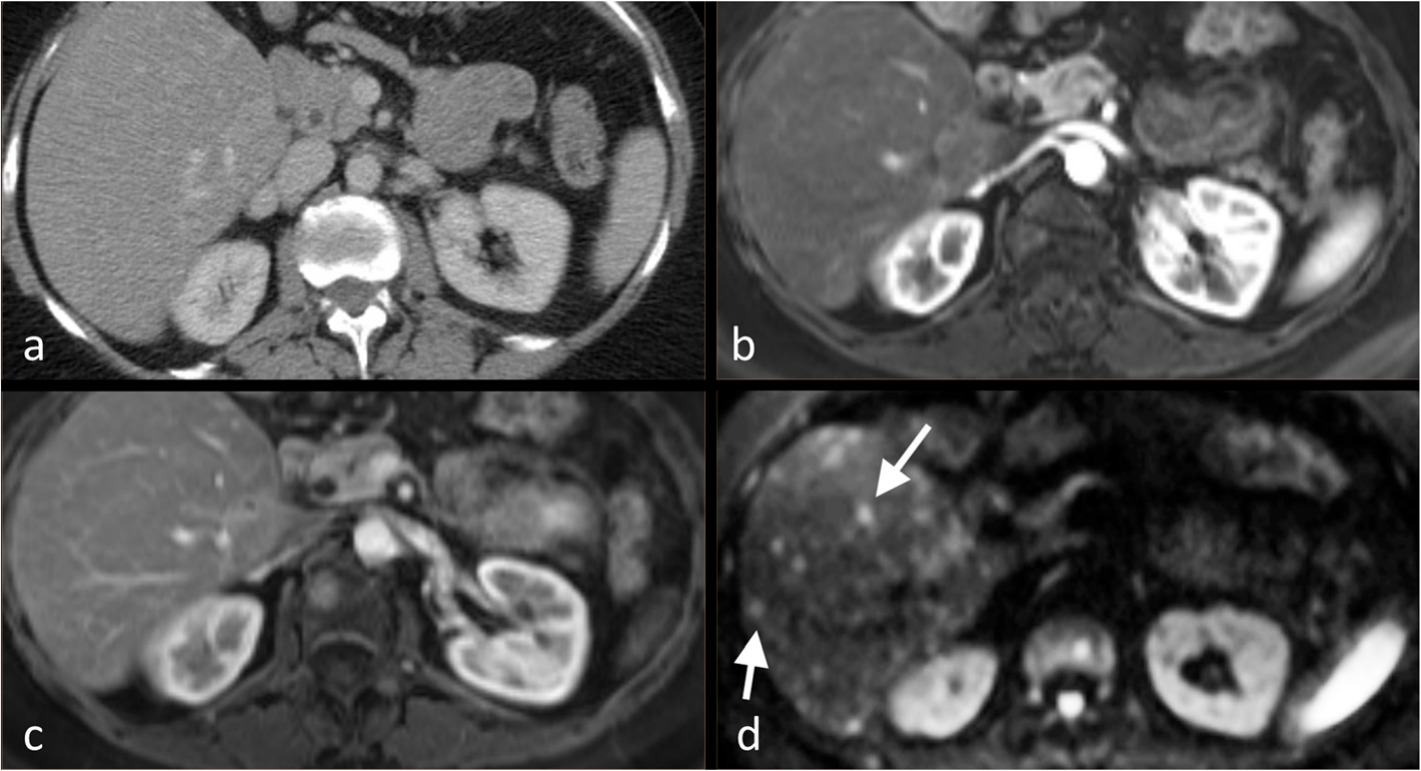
Isodense liver metastases not visualised on CT identified using MRI. Images from a portal venous phase CT (a), arterial (b) and portal venous (c) phase T1-W post-contrast MRI and b-750 diffusion-weighted MRI (d) through the liver in a 46-year-old woman with breast cancer and deranged liver function. The liver appears normal on both CT and T1-W post-contrast MRI but multiple high signal foci are present throughout the liver (white arrows) on the diffusion-weighted sequence in keeping with diffuse liver metastases
